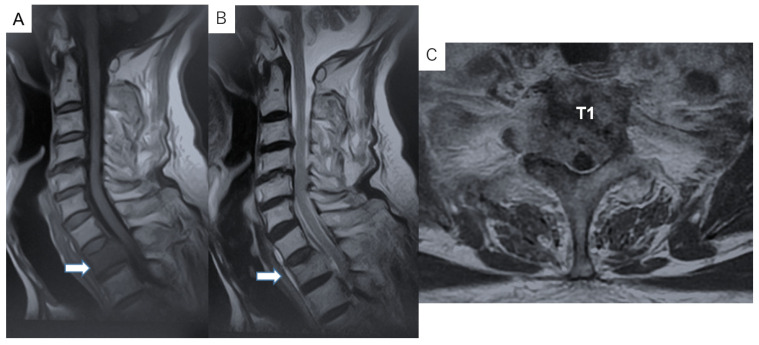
Magnetic resonance imaging (MRI) shows spinal cord compression by an epidural mass at the T1 level: (A) T1-weighted midsagittal image; (B) T2-weighted midsagittal image; and (C) T2-weighted axial image at the T1 vertebral level.
